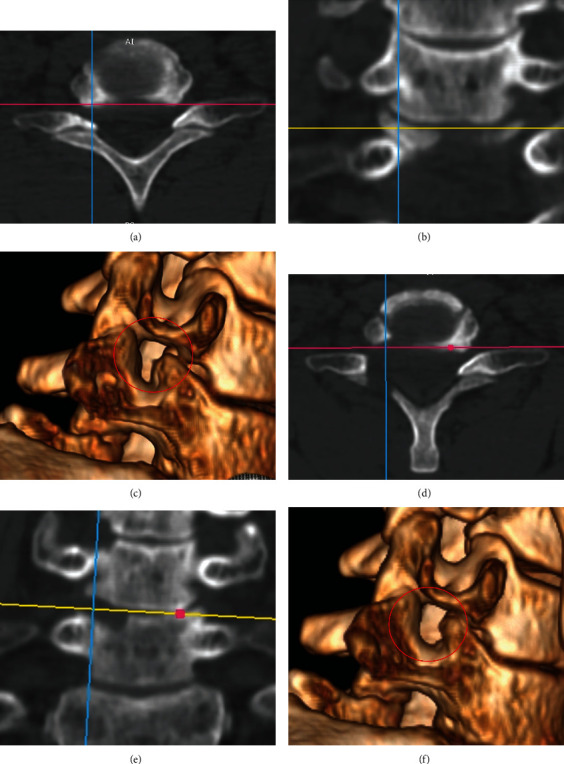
Comparison of CT images before and after decompression from the inferior of the exiting root at the C6-7 level. Preoperative images: (a) axial view, (b) coronal view, and (c) reconstruction image. Postoperative images: (d) axial view, (e) coronal view, and (f) reconstruction image.
